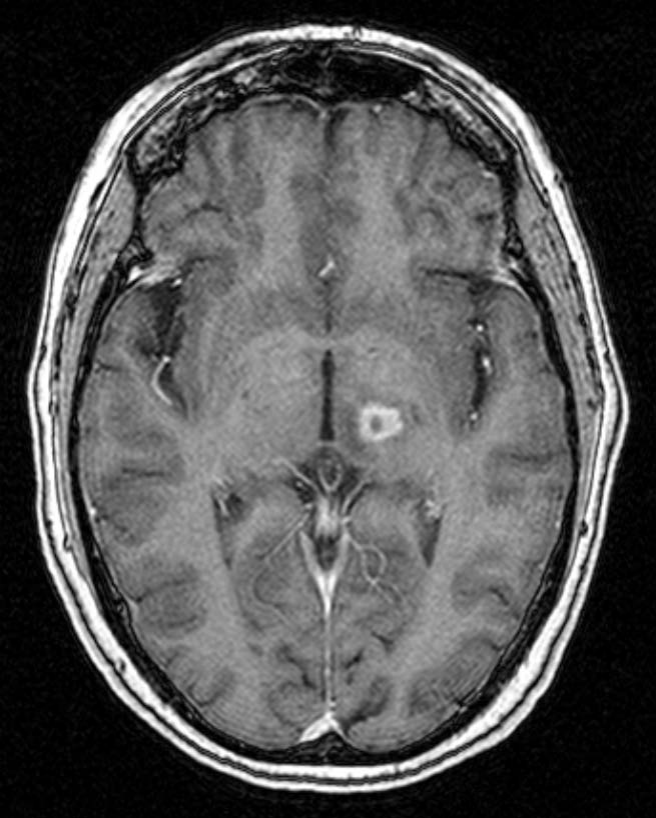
MRI 1 year after radiosurgery.
